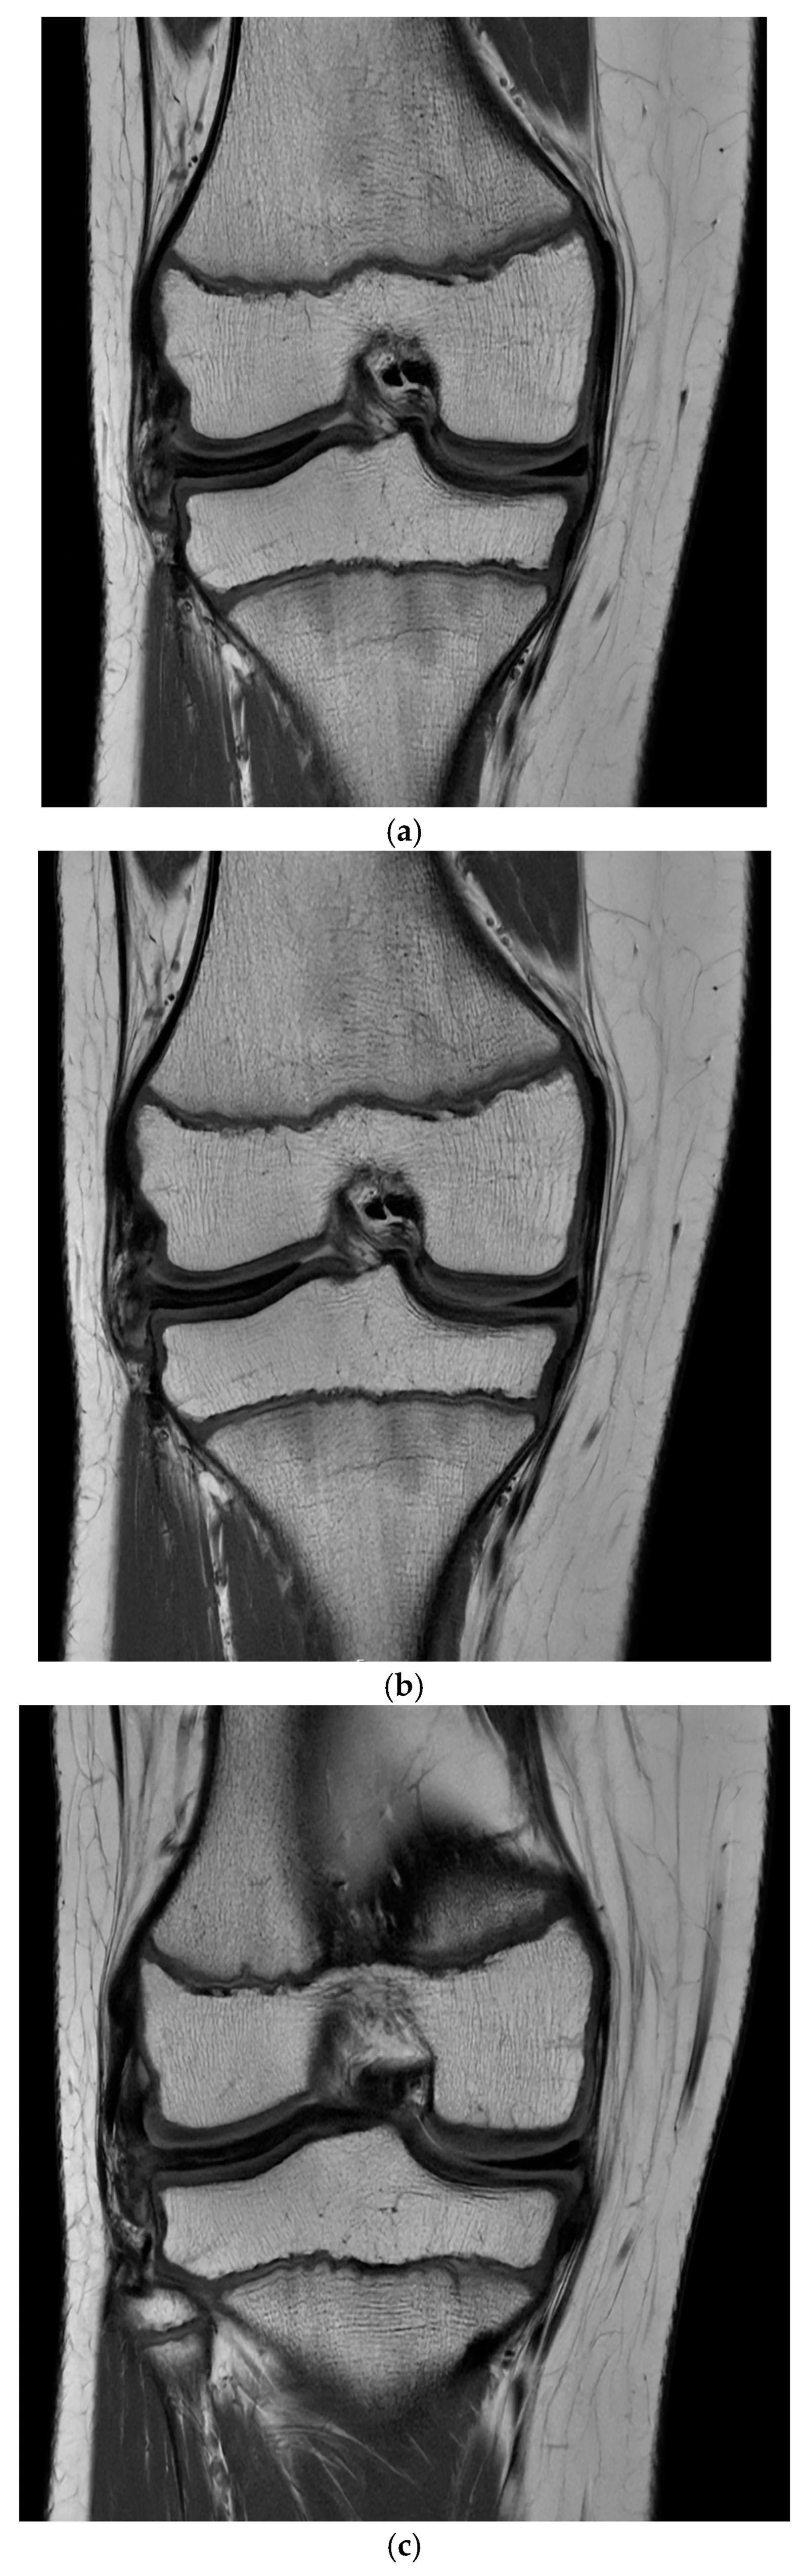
Three consecutive coronal MR images demonstrating the “Bow-tie” sign in the discoid meniscus and a horizontal tear in the meniscus body, with (a) being the most anterior, (b) an intermediate slice showing continuity of the meniscal body, and (c) being the most posterior slice.
